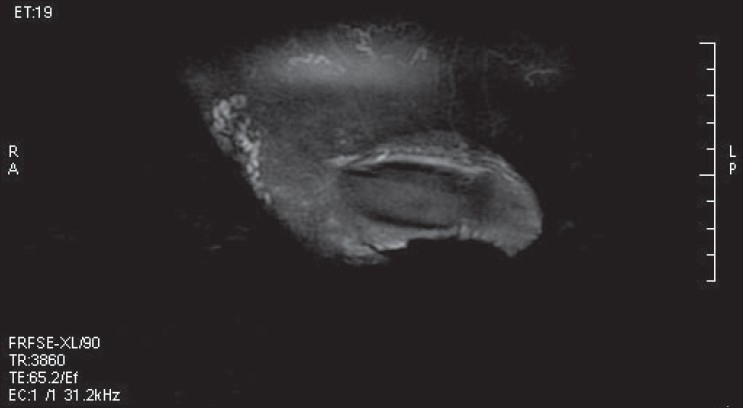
MRI scan of the penis showing lymphangiectasia
